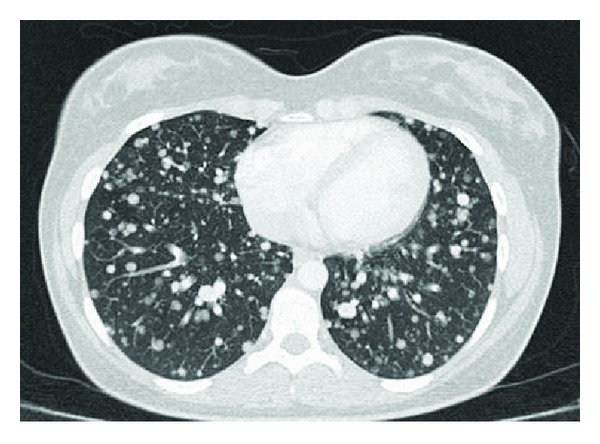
In thoracic CT, multiple atypically located nodule formations are seen in bilateral lungs.
